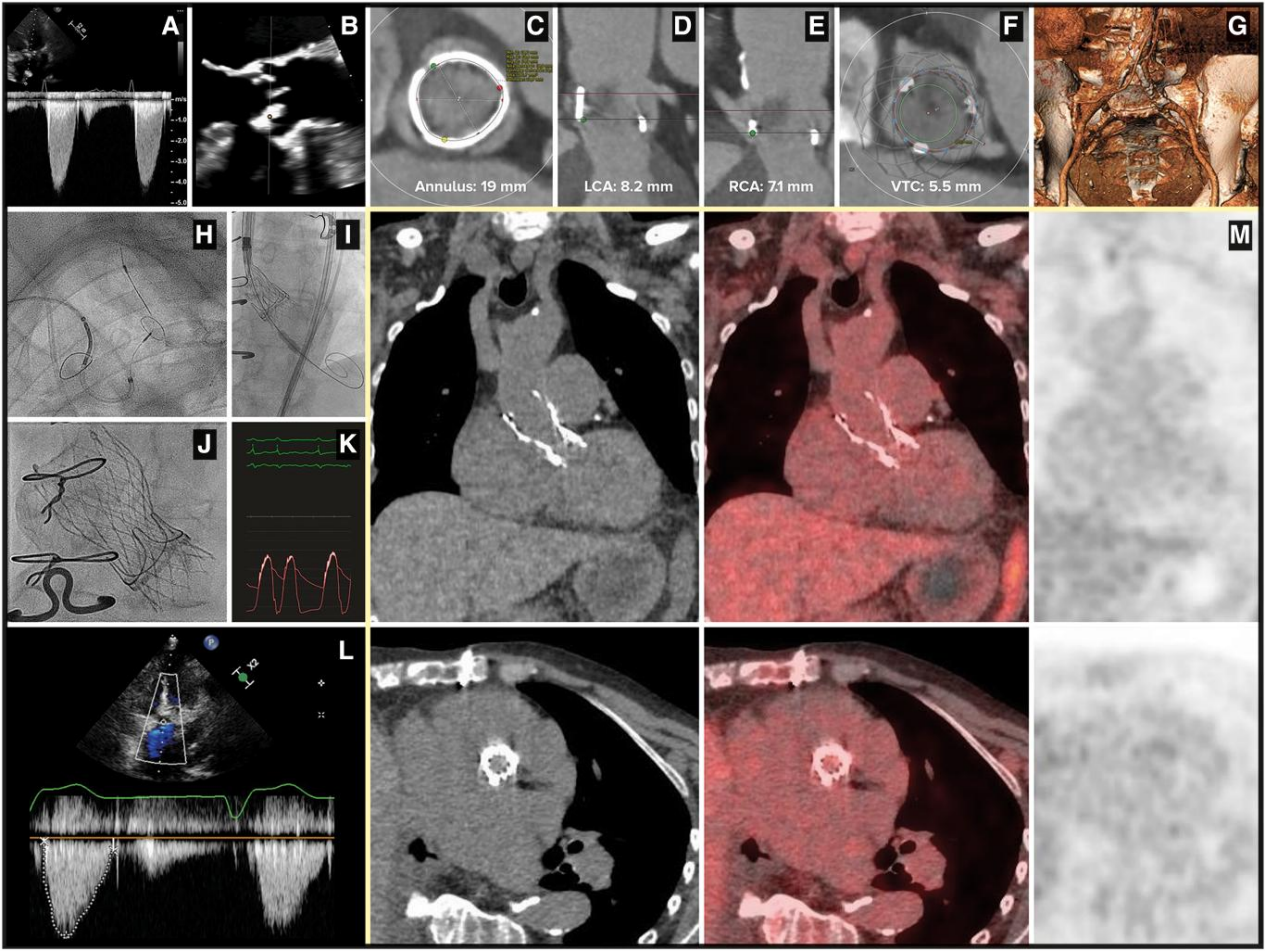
(*A*) Transvalvular aortic peak gradient obtained by transthoracic echocardiography. (*B*) Vegetation on the right cusp of the bioprosthetic valve at transoesophageal echocardiography. (*C*) Aortic annulus dimensions by CT-scan. (*D*) Distance between annulus and left coronary artery (LCA) ostium measured by CT-scan at 8.2 mm. (*E*) Distance between annulus and right coronary artery (RCA) ostium measured by CT-scan at 7.1 mm. (*F*) Virtual distance between transcatheter valve and coronary ostium (VTC) measured by CT-scan at 5.5 mm. (*G*) Femoral vascular access by CT-scan. (*H*) A cerebral protection device Sentinel deployed by the right radial access. (*I*) Fluoroscopic aspect of the Evolut-R 23 mm deployed in a Magna Ease 21 mm using the valve-in-valve technique. (*J*) Fluoroscopic aspect of the Evolut-R 23 mm implanted in the Magna Ease 21 mm. (*K*) Haemodynamic invasive transvalvular gradient after TAVI. (*L*) Transvalvular aortic gradient at follow-up after TAVI assessed by transthoracic echocardiography. (*M*) Absence of abnormal F-18-fluorodeoxyglucose uptake at PET-CT at follow-up after TAVI.
